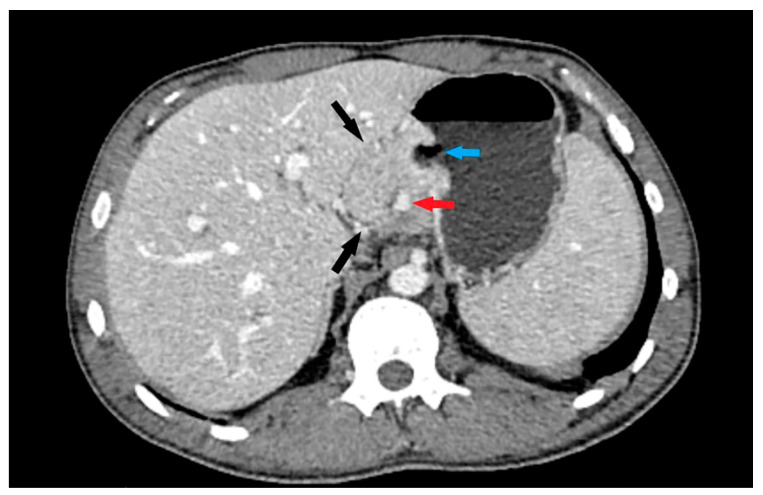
Axial CT scan shows exophytic tumor growth into gastro-hepatic ligament (black arrows) with presence of intralesional vascular structures (red arrow) and mucosal defect: umbilication (blue arrow). Tumor involves corpus region of the stomach.
